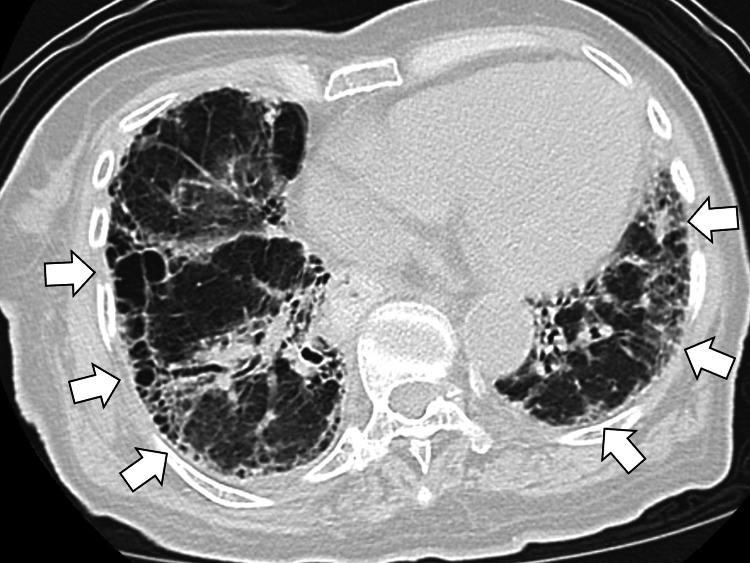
Chest computed tomography revealing a pattern consistent with nonspecific interstitial pneumonia, including reticular and ground-glass opacities along the bronchovascular bundles and honeycombing at the lung bases (white arrows)
